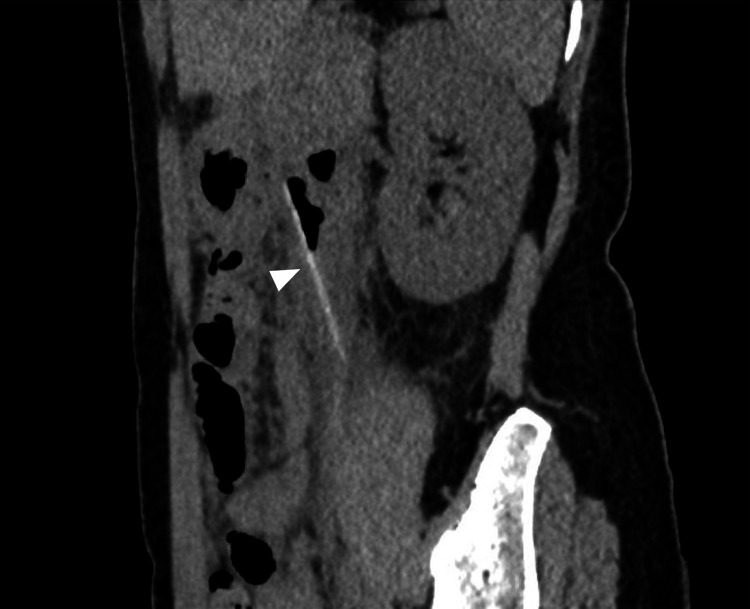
Abdominal contrast-enhanced computed tomography An abdominal contrast-enhanced computed tomography scan showed a 6 cm linear opacity in the jejunum, indicative of foreign body penetration near the ligament of Treitz without free air. The arrow also indicates the foreign body.
